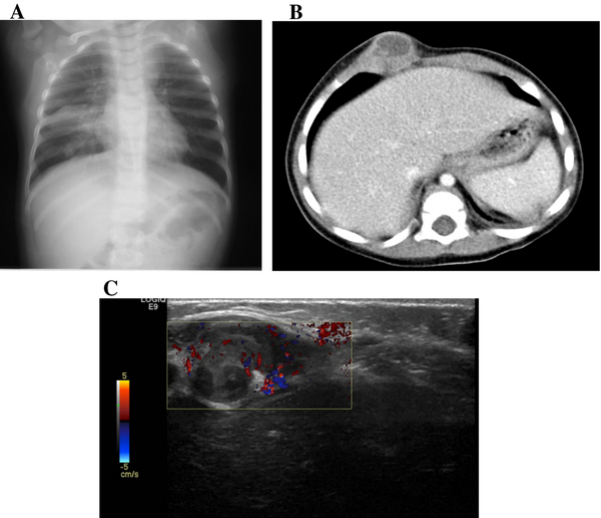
a: chest x-Ray showing right lung hypodiaphany; b: Computed Tomography scan performed during the first hospitalization in Romania showing a ring-enhanced, well-circumscribed abscess adherent to the cartilagean rib; c: ultrasonography study performed at our institution, showing a lesion with peripheral area of hypervascularization.
